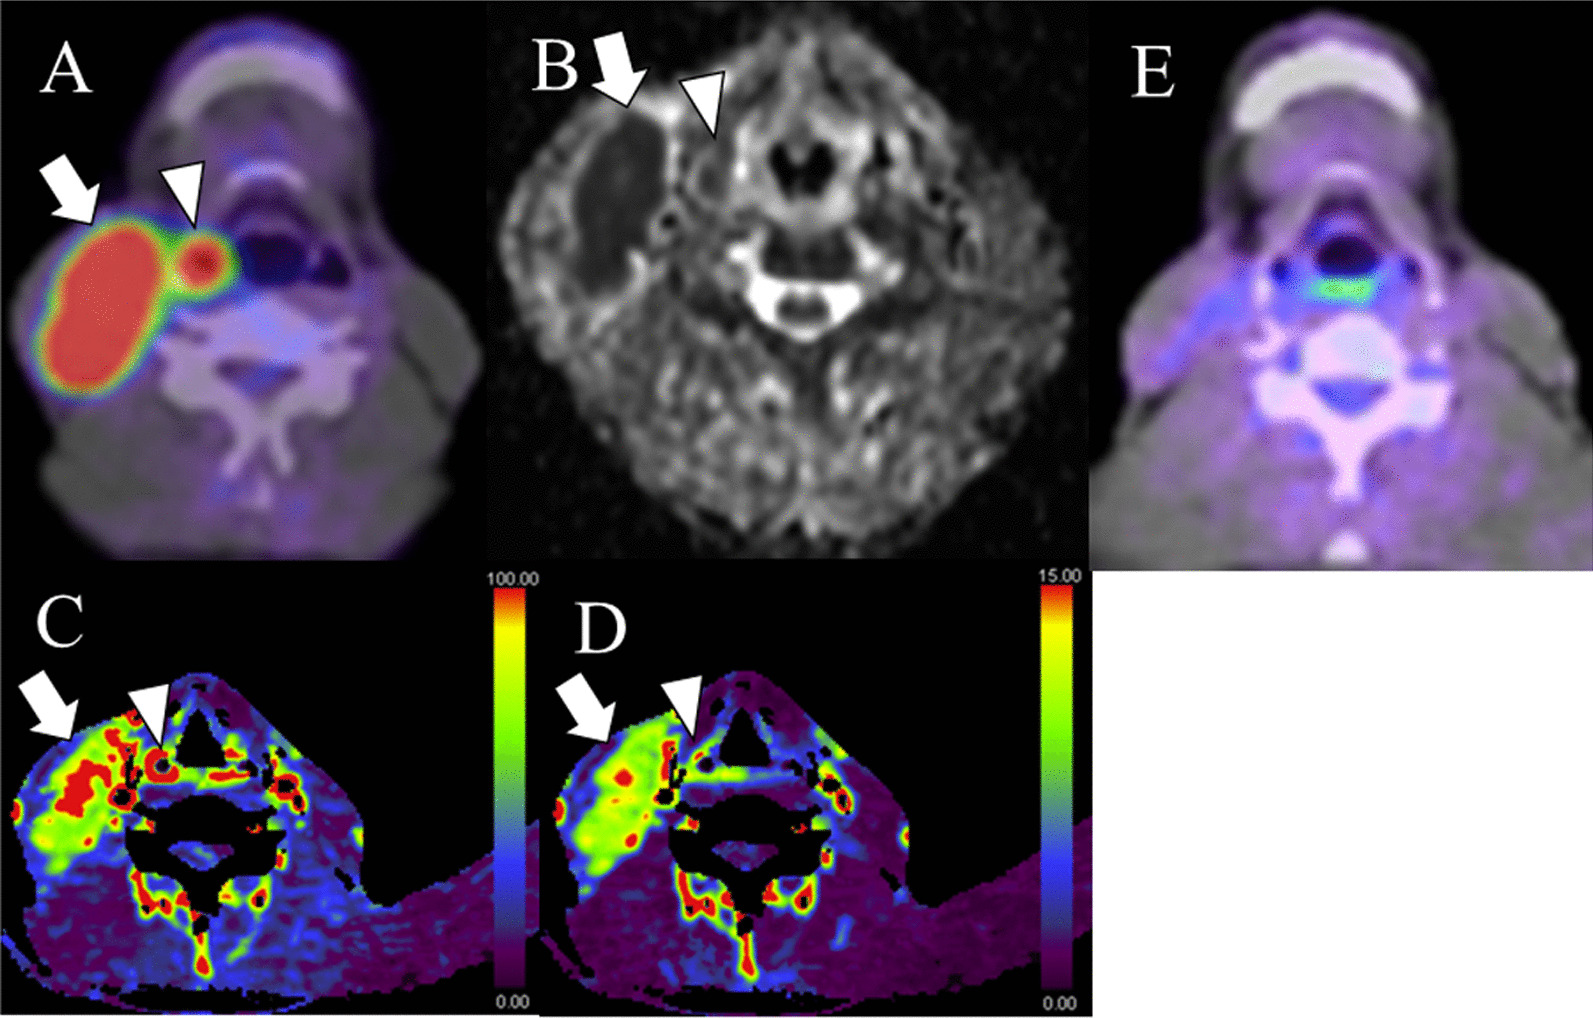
A 63-year-old man with right oropharyngeal squamous cell carcinoma (arrowhead) as well as enlarged lymph node (arrow) in level II on right side. a Pretreatment PET-CT. b The corresponding ADC map. c The corresponding BF map. d The corresponding BV map. The primary tumor showed BF value of 126.7 mL/min/100 mL, BV value of 12.3 mL/100 mL, and skewness of ADC of 0.71. The metastatic node showed BF value of 119.4 mL/min/100 mL, BV value of 11.1 mL/100 mL, and skewness of ADC of 0.69. e PET-CT at 12 weeks post-treatment demonstrates the persistence of mild ^18^F-FDG uptake in the metastatic lymph node site. However, clinical and imaging follow up did not disclose any recurrence of primary tumor and lymph node
